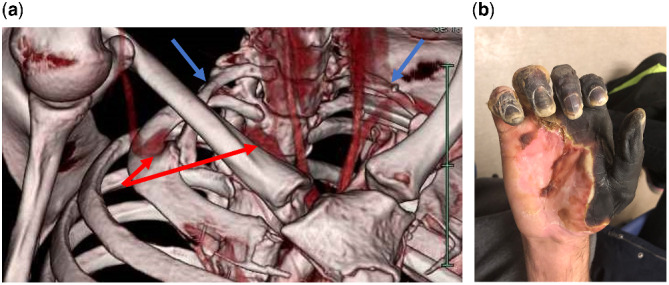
Radiological and clinical findings of Case 1 (A) CT angiogram of the right upper limb showing bilateral cervical ribs (blue arrows) and compression of the right subclavian artery in the abduction position (red arrows). The right subclavian artery was found to be patent in the neutral position, with mild dilatation distal to the cervical rib. (B) Dry necrosis of the right hand 6 weeks postoperatively. The old scar on the volar side of the wrist from penetrating trauma at age of 14 years is demonstrated.
